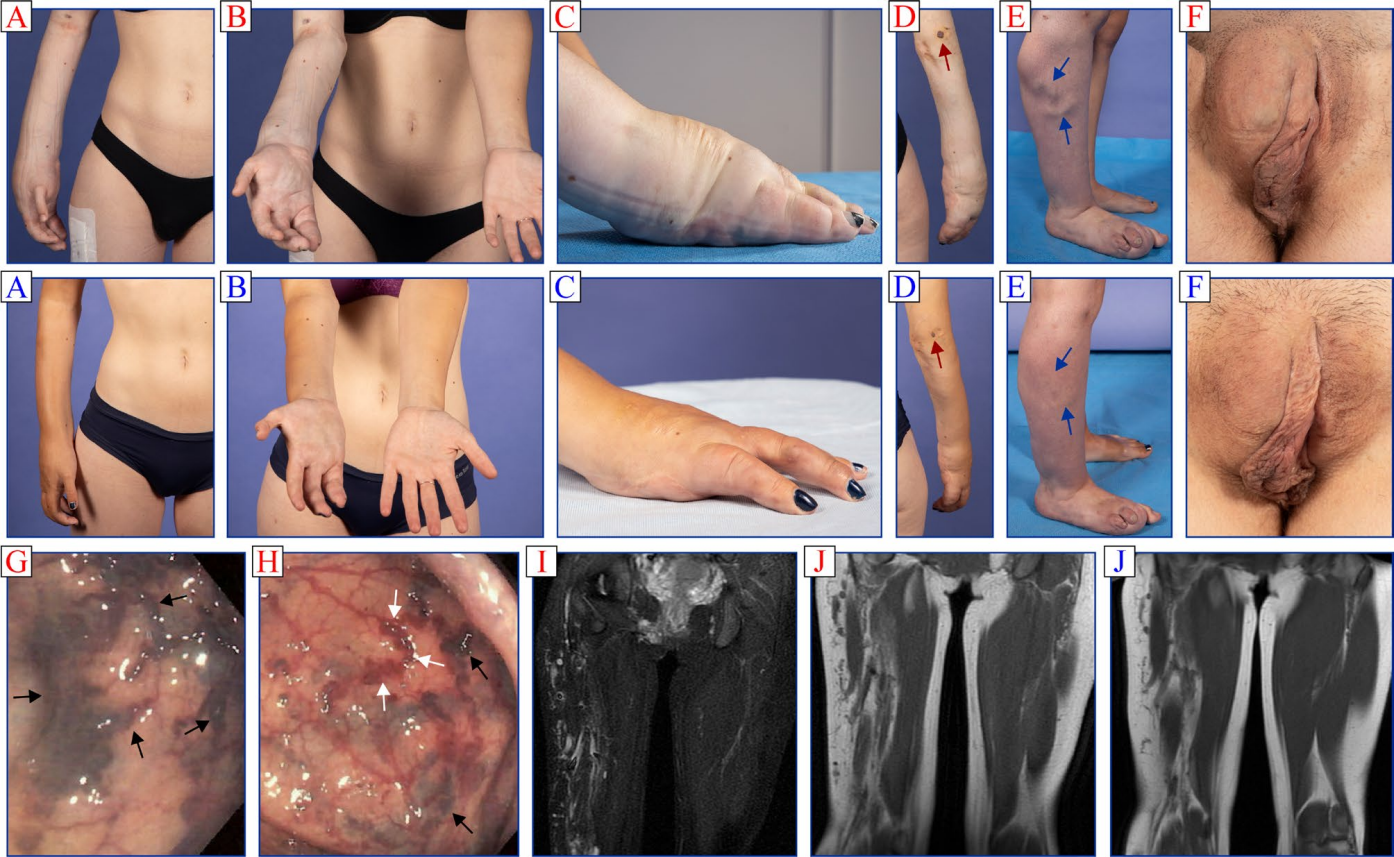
Clinical manifestations at baseline (A to F and G to J in red) and after 2 months on alpelisib (A to F and J in blue). A–F in red, external appearance before treatment. Right distal arm is seen to be severely enlarged (panels A to D) with an epidermal nevus near the elbow (red arrows in panels D), right distal leg to be moderately enlarged (panel E) with a large venous ectasia (blue arrows in the same panels), and upper outer lip of vagina to harbor a large venous mass (panels F). Mild hypertrichosis was also present in the distal hypertrophied extremities (barely visible on the pictures shown). A–F in blue, external appearance during treatment. Areas of overgrowth and epidermal nevus have decreased by more than 50% (panels A to E) and so has hypertrichosis (barely visible on the pictures shown), while the venous ectasia and perineal mass have almost completely disappeared (panels E and F). G to J in red internal vascular malformations before treatment. Appearance of sigmoid (panel G) and colon at the splenic angle (panel H) based on endoscopic images. The mucosae are seen to harbor multiple vascular ectasias (black arrows in panels G and H) and vascular tortuosities (white arrows in panel H). Appearance of lower body based on MRI STIR- or T1-weighted images (panels I and J). Right leg, uterus and bladder are all seen to harbor multiple venous malformations. Note that the right hand and right foot were both affected by moderate adipose overgrowth (not shown). J in blue internal vascular malformations during treatment. Vascular malformations have decreased by more than 60% based on MRI T1-weighted images. A control colonoscopy has still to be conducted
